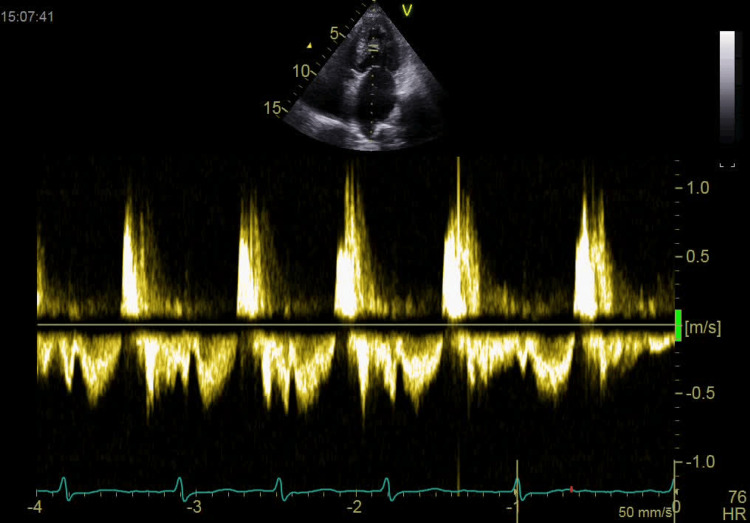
Continuous Doppler of grading diastolic intraventricular obstruction caused by the non-valvular papillary fibroelastoma.
